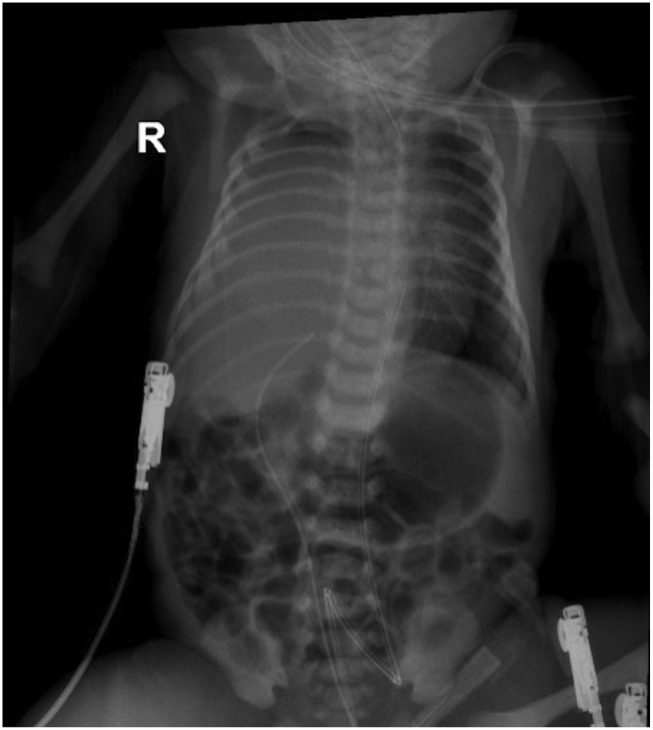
A chest radiograph was performed on day 1 after birth, which revealed complete opacification of the right hemithorax.
